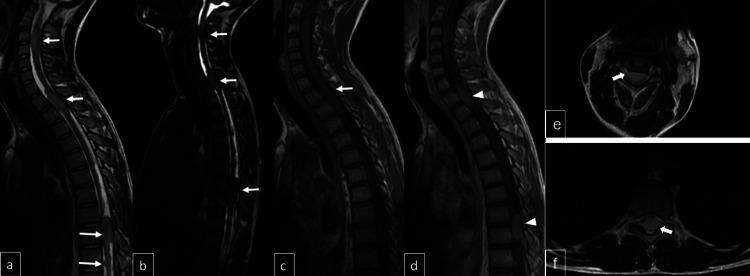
Sagittal T2-weighted (a), FIESTA (b), T1-weighted (c), and post-contrast T1-weighted (d) images demonstrate intradural nodular lesions within the spinal canal (arrows) with contrast enhancement (arrowheads), while axial T2-weighted images (e and f) confirm their intradural extramedullary localization (arrows). FIESTA: Fast Imaging Employing Steady-State Acquisition
